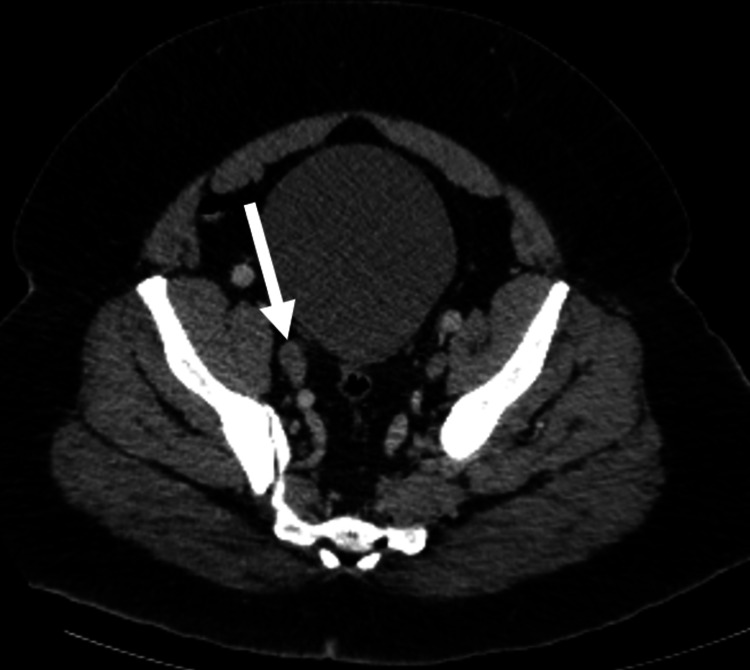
Axial CT scan of the pelvic region A mildly enlarged 12.6 mm right-sided pelvic lymph node is seen (white arrow).
